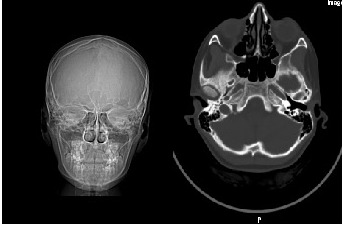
Cerebral CT scan with coronal and axial views showing normal bone structure, with no fluid accumulation within sinuses, no periorbital or cerebral inflammation
